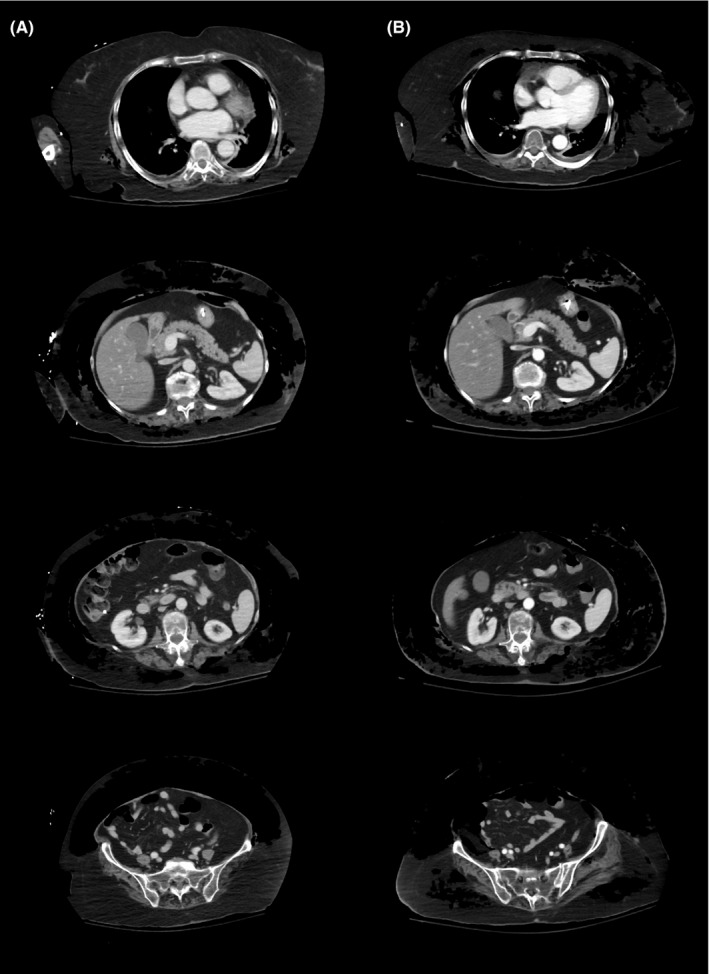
Initial (A) and follow‐up (B) computed tomography scans of the patient, showing worsening subcutaneous emphysema
